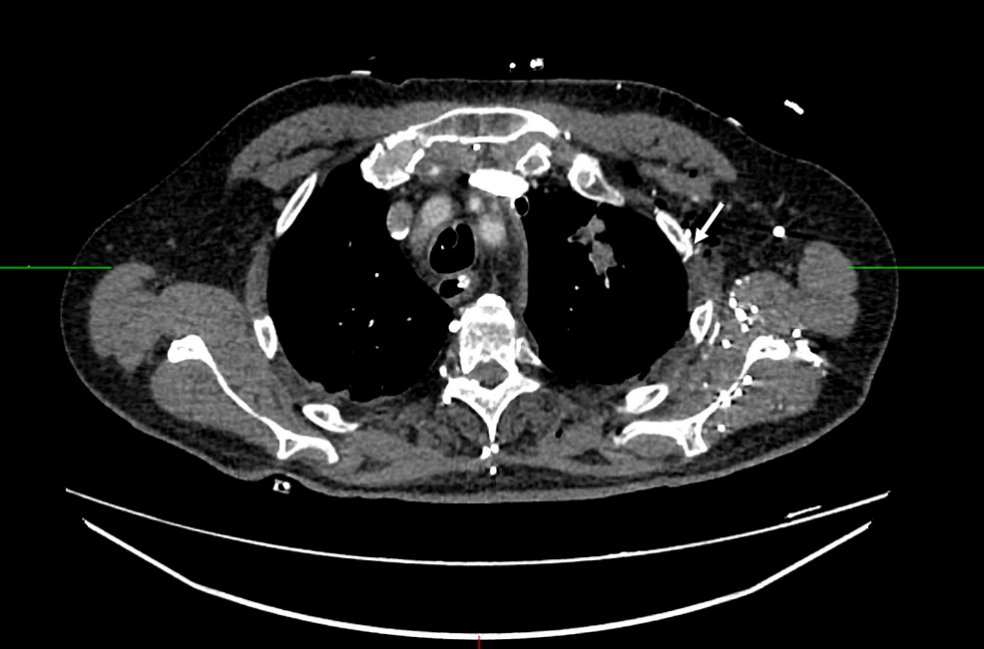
Contrast-enhanced computed tomography showed small pleural hemorrhage and third and fourth rib fractures (white arrow).
